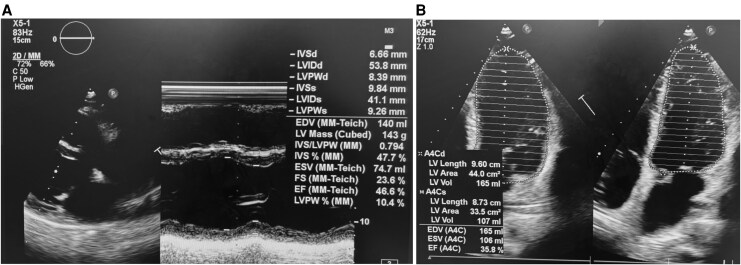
(A)* Parasternal long-axis view with M-mode shows marked left ventricular dilatation with increased end-diastolic and end-systolic dimensions. *(B)* Apical four-chamber view demonstrates the left ventricular ejection fraction was approximately 35.8% by Simpson, consistent with severe dilated cardiomyopathy.
